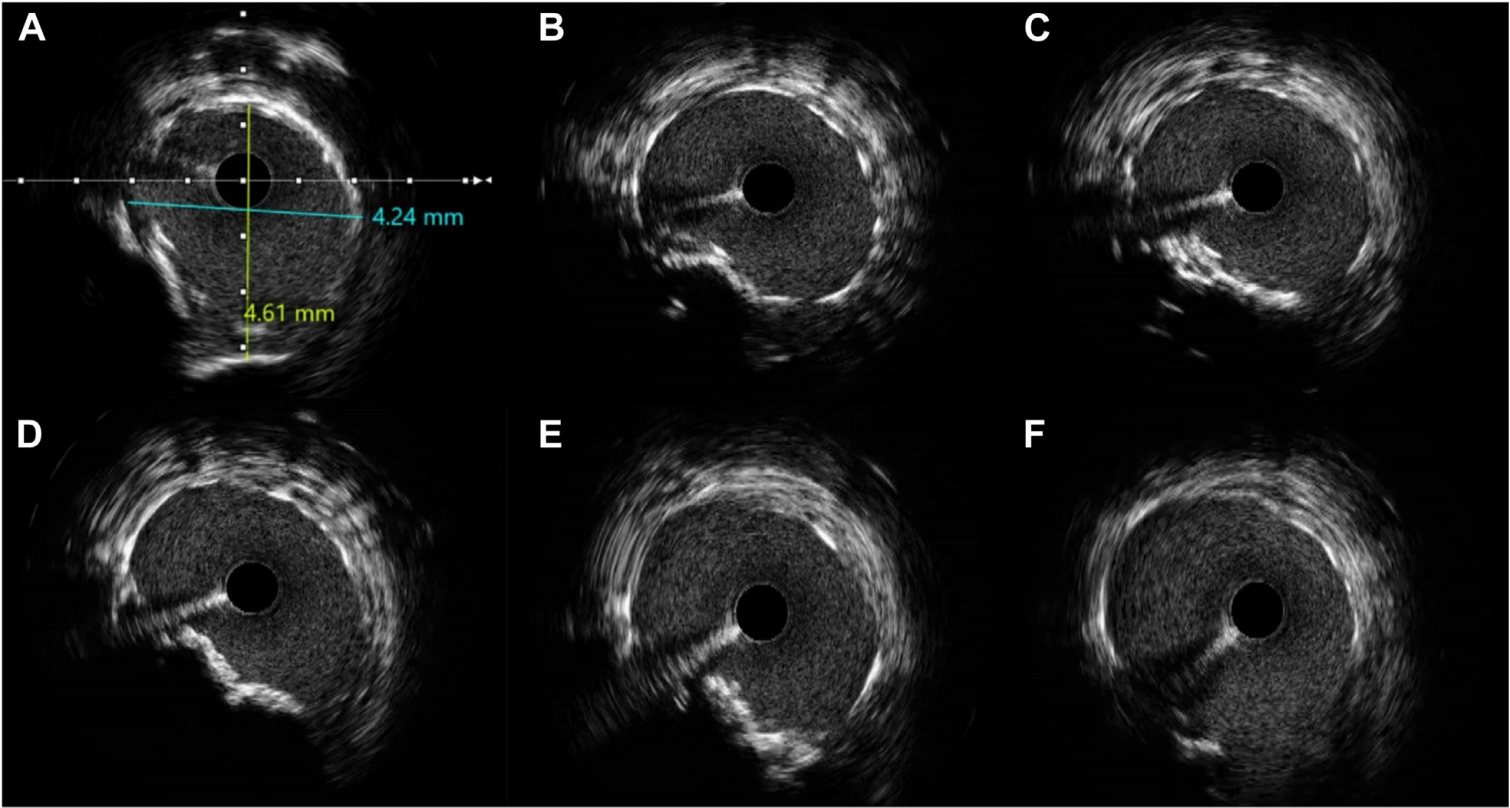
Intravascular Ultrasound-Guided Left Main Artery Stenting (A) Intravascular ultrasound image of left main artery stent with a measurement of 4.2 mm × 4.6 mm, guided post-dilatation by a 5-mm noncompliant balloon. (B to F) Sequential intravascular ultrasound images post-stent optimization reveal good stent expansion and proper apposition of left main artery stent.
